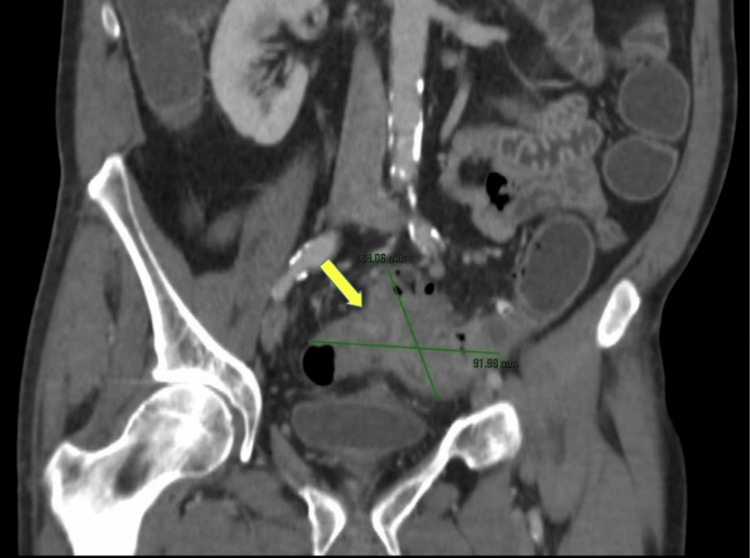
Coronal section of the abdominal CT scan showing the dimensions (annotations) of the mass in the sigmoid colon (arrow). CT: Computed tomography.
